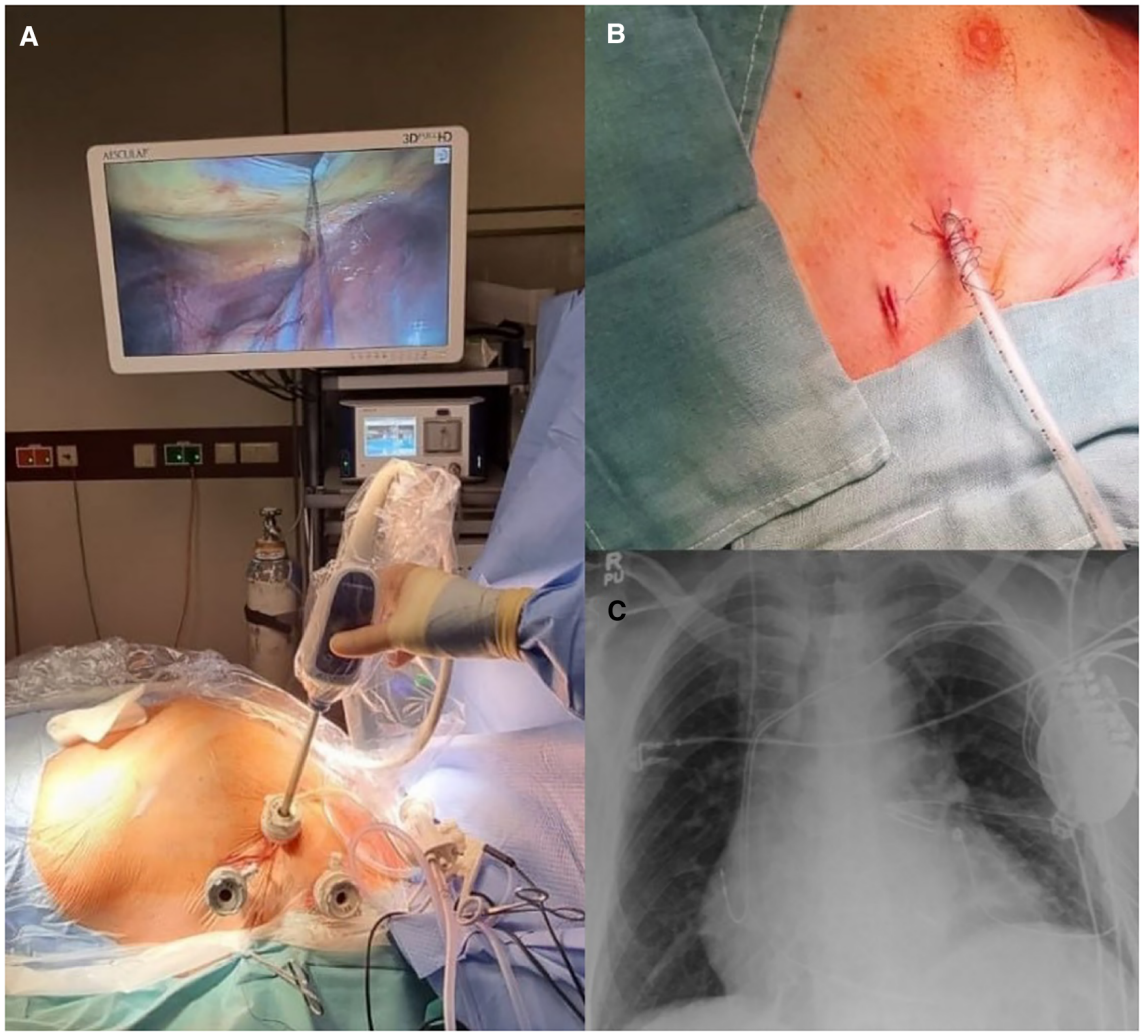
(A) Intraoperative picture showing the three-port access and the 3D videoscopic guidance. (B) Postoperative state after wound closure. (C) Postoperative chest x-ray showing the CRT-D device with the epicardial LV lead and the AtriClip PRO2® device.
